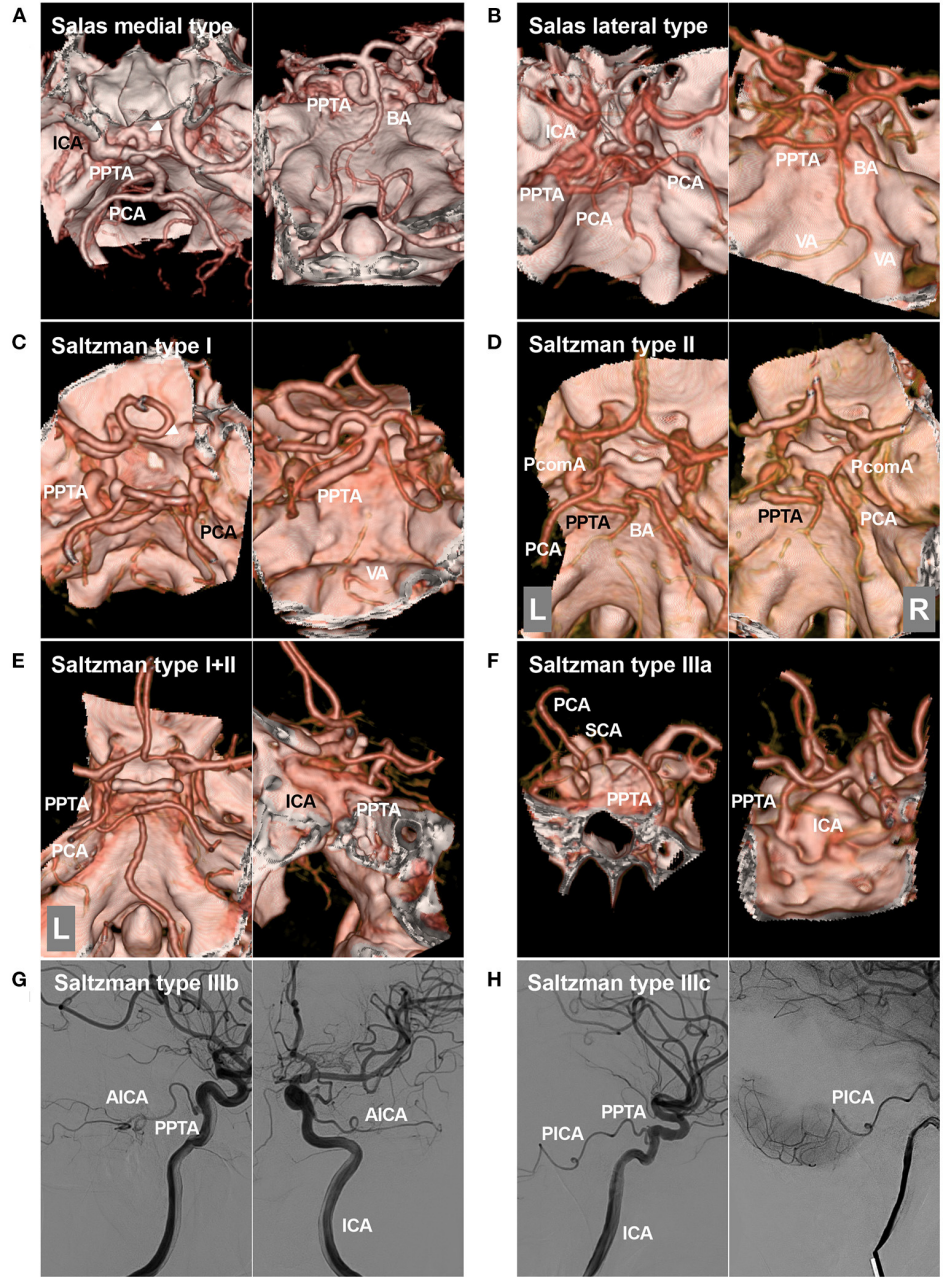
Salas and Saltzman classifications. (A): Salas medial (sphenoidal) type. Left, CTA showing the PPTA arising from the ICA, running medially into the sella (arrowhead), and then crossing the dorsum sellae. Right: CTA showing the PPTA connecting to the BA. (B): Salas lateral (petrosal) type. Left, CTA showing the PPTA arising from the ICA, running laterally, and then crossing the cavernous sinus. Right: CTA showing the PPTA connecting with the BA. (C): Saltzman type I. Left, CTA showing the PPTA arising from the ICA. Right: CTA showing the PPTA connecting with the BA. In this case, the PPTA is strong and hyperplastic, and the PcomAs and vertebrobasilar artery are hypoplastic. (D): Saltzman type II. Left, CTA showing the left PPTA and the left fetal-type PCA; Right, CTA showing the right PCA receiving its blood supply from a patent PcomA. The proximal BA is well developed. (E): Saltzman combined type (I+II). Left, CTA showing that the left PCA is fetal-type and not connected with the BA, the right PCA originates from the BA, and no PcomA can be seen; Right: CTA showing that the PPTA arises from the left ICA. The vertebrobasilar arteries are normally developed. (F): CTA showing a type IIIa PPTA arising from the ICA and then extending to the opposite SCA. (G): DSA of the ICA showing a type IIIb PPTA arising from the ICA and then extending into the AICA. (H): DSA of the ICA showing a type IIIc PPTA arising from the ICA and then extending into the PICA. AICA, anterior inferior cerebellar artery; BA, basilar artery; CTA, computed tomography angiography; DSA, digital subtraction angiography; ICA, internal carotid artery; L, left; PCA, posterior cerebral artery; PcomA, posterior communicating artery; PICA, posterior inferior cerebellar artery; PPTA, persistent primitive trigeminal artery; R, right; SCA, superior cerebellar artery; VA, vertebral artery.
